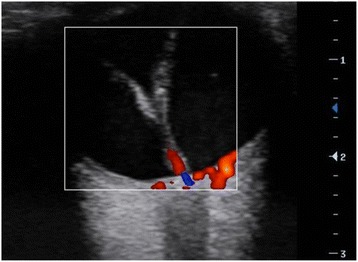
ocular ultrasound - a V-shaped membrane attached to the optic nerve head, indicative of a total retinal detachment
